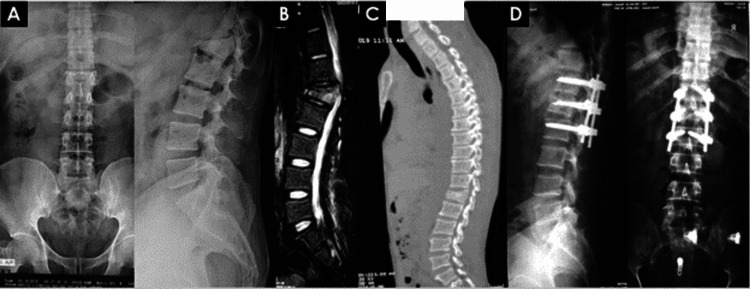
42-year-old male with burst #L1 with ASIA-C neurology, treated with short-segment posterior fixation including fractured vertebra without fusion (Patient 2). The treatment for a 42-year-old male with burst #L1 and ASIA-C neurology involved short-segment posterior fixation, which included the fractured vertebra (Figure 2) without fusion. Panels A–D demonstrate the following: A: Pre-operative X-ray, lumbo-sacral spine (AP and lateral view) B: MRI of LS spine (T2 sagittal section) C: CT scan of LS spine (sagittal section) D: Final follow-up at three years Image Credit: Sharif Jonayed
